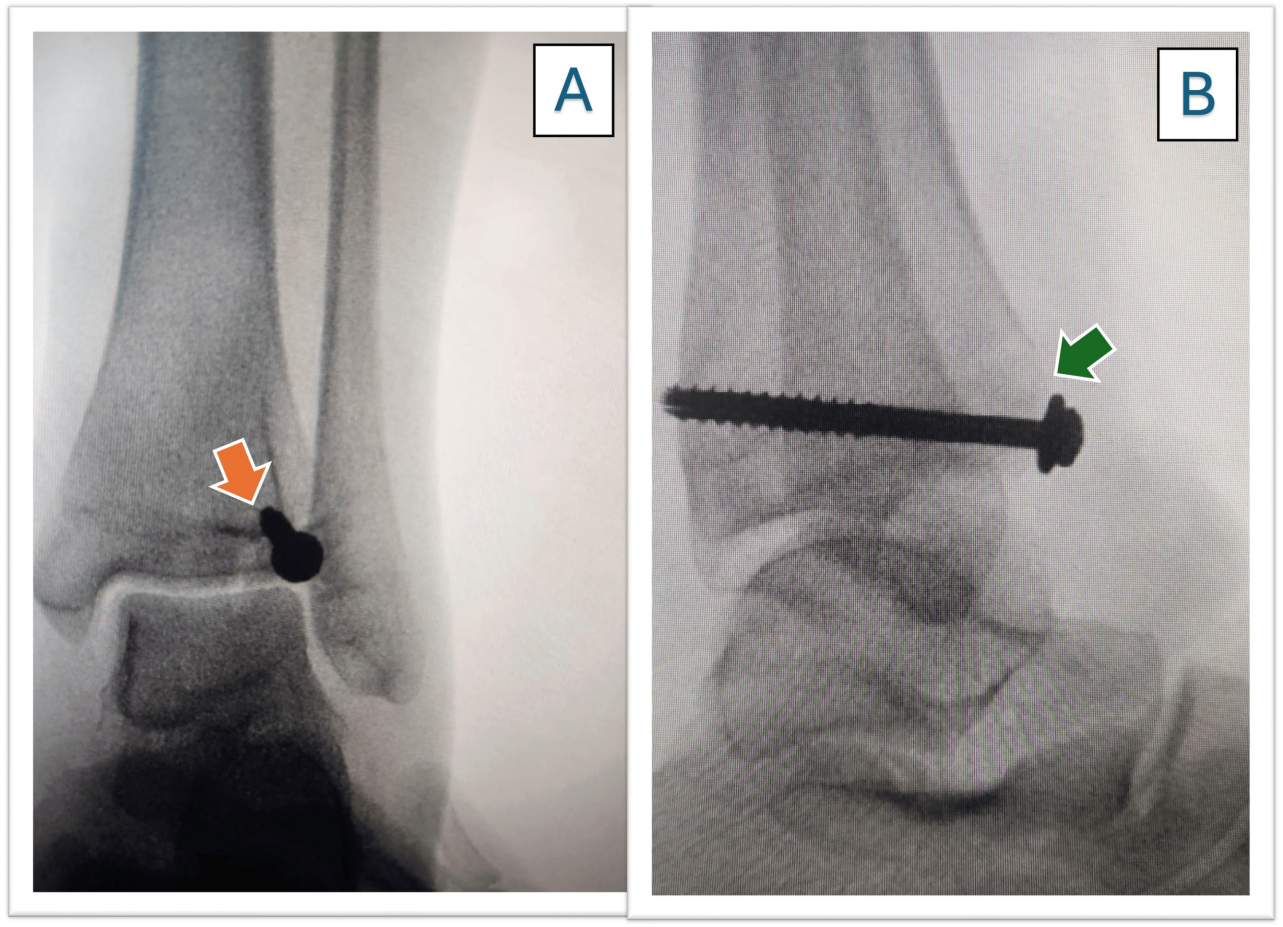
Anterioposterior and lateral postoperative radiographs. A) The orange arrow indicates the position of the cannulated screw in the anteroposterior view, showing an acceptable reduction with a minimal residual articular gap. B) the green arrow shows the lateral view, where the alignment is satisfactory despite the presence of a small articular gap.
